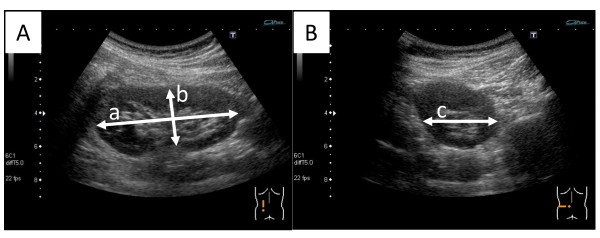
Measurement of the kidney size by ultrasound. (A) We first select an image where the renal hilum is clearly observed and the area of longitudinal plane is at the maximum. In this image, the major axis (line "a") and minor axis (line "b") are measured. The line "b" should be vertical to the major axis and pass through the center of the renal hilum. (B) Next, we select the maximum transverse image, which is almost a circle, and passes through the center of the renal hilum. In this image, the transverse axis (line "c") is measured.
